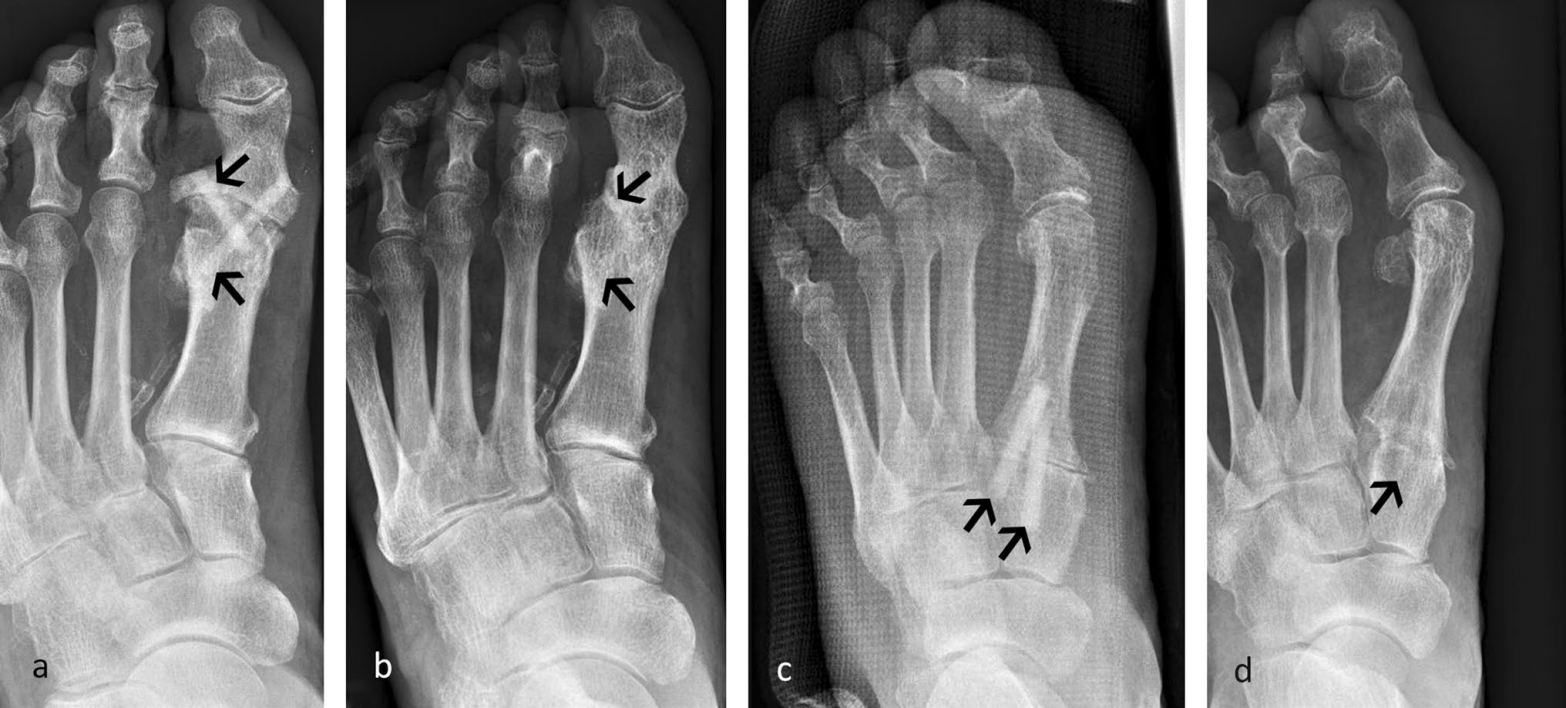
Examples of the application of the allogeneic screw in foot surgery. Radiographs showing the incorporation of the transplant used for an MTP I arthrodesis of a patient with type 2 diabetes and peripheral arterial occlusive disease a on the day of surgery, b 20 months after surgery and radiology showing the incorporation of the allogeneic screw used for a Lapidus arthrodesis c 6 weeks and d 12 months after surgery. Transplants are marked by arrows
